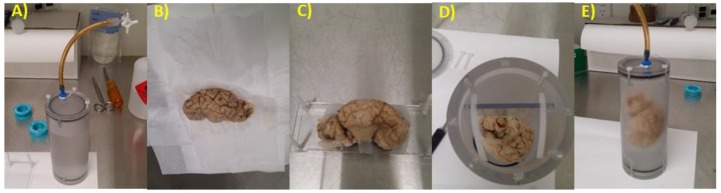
Brain tissue preparation for MRI acquisition. (A) The custom-made chamber has a tight seal which is secured with plastic screws. The lid has a tube attached to a 3-way tap to allow de-gassing. (B) The brain is patted dry with Kimwipes^®^ (Kimberly-Clark), until no more water is present on the tissue. (C) The brain is gently taped onto the platform, so that it will not move. (D) The platform should be a secure fit in the chamber. (E) The lid is then placed back on securely, and the brain is fully immersed in Fluorinert, and de-gassing can commence.
